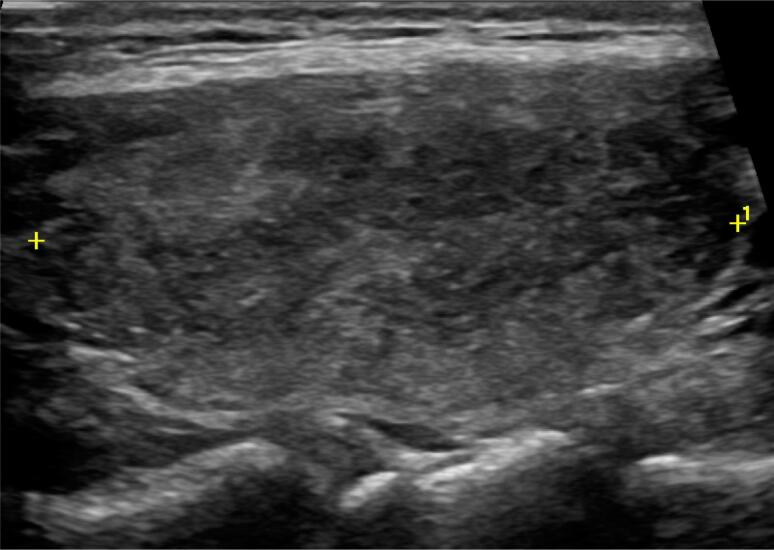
Thyroid ultrasound: diffuse hypoechoic left thyroid lobe.
